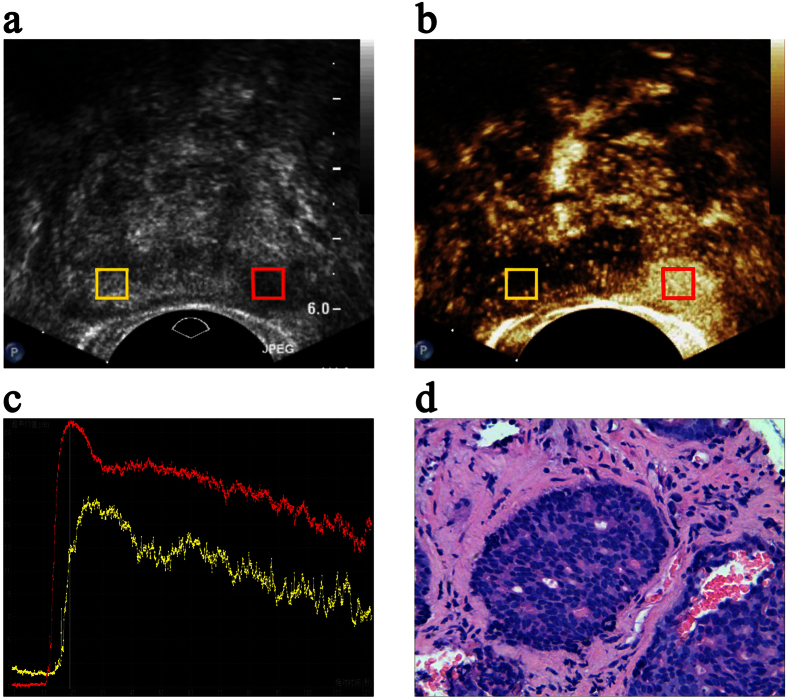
The region of interest (ROI) settings, time-intensity curve (TIC) analysis and oncological outcomes. Transverse (a) greyscale and (b) contrast-enhanced transrectal ultrasound mapping of the prostate in a 67-year-old patient. The red box indicates the ROI placed in the nodule lesion, and the yellow box indicates the corresponding ROI placed in the normal peripheral zone (PZ). (c) The red and yellow lines in the TICs represent the perfusion characteristics of the ROIs of the nodules and the normal PZ, respectively. (d) The oncological outcomes following biopsy show an adenocarcinoma.
